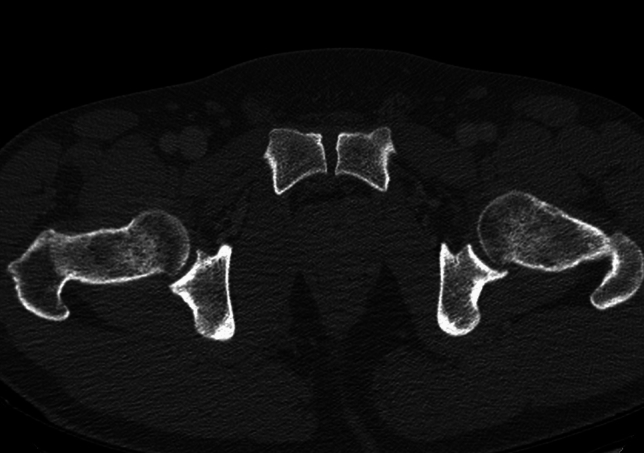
Axial CT view of a pelvis with an uninjured pubic symphysis with anterior physiological widening compared to the posterior symphyseal part
